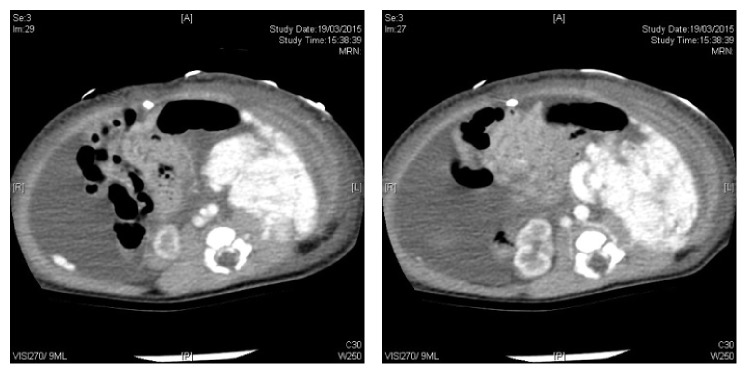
Postnatal CT scan showed a large multilobulated enhancing mass.
